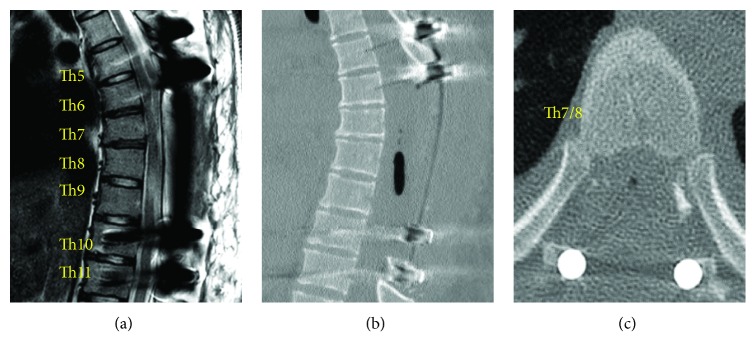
Thoracic spine radiological findings post Th5-Th11 posterior spinal fusion. Postoperative MRI showed the dura matter swollen (sagittal view (a)), while CT demonstrated the total resection of these extremely ossified components (sagittal view (b), axial view in Th7/8 level (c)).
